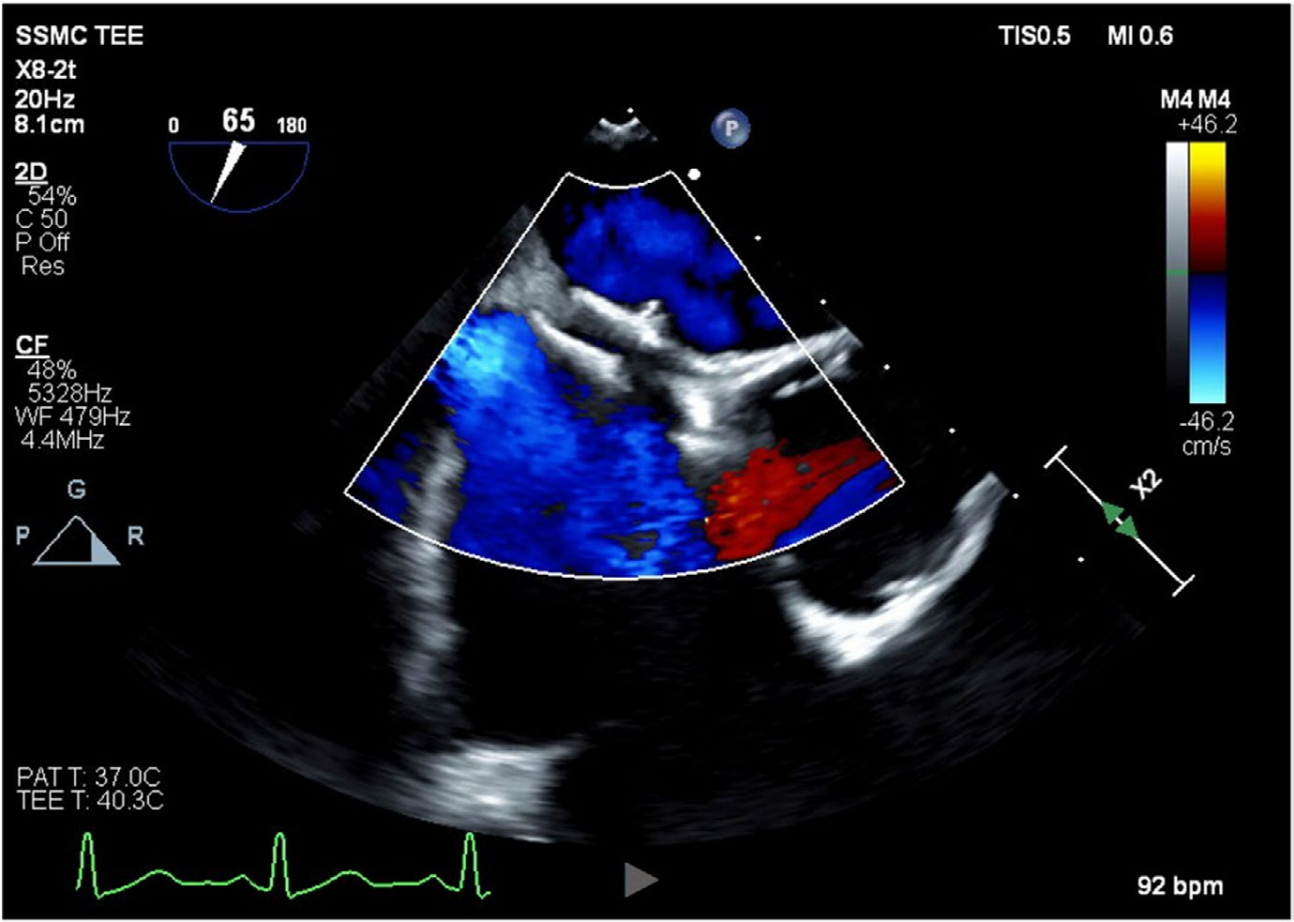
Intraoperative transesophageal echocardiography prior to atriotomy showed complete closure of the patent foramen ovale, with a nickel‐based Amplatzer occlusion device in situ.
